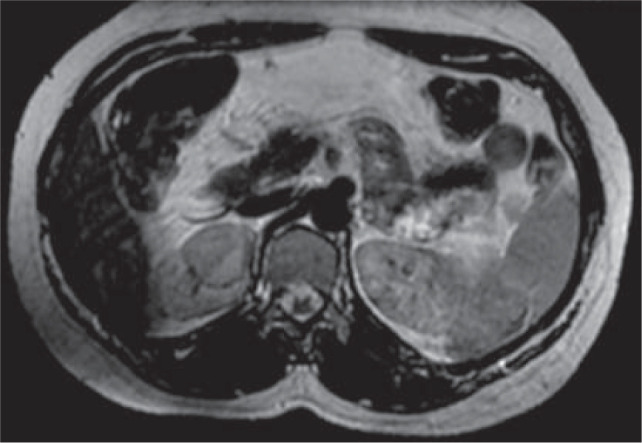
Magnetic resonance imaging: T2 isointense to intermediate well-defined lesion in the right kidney.
